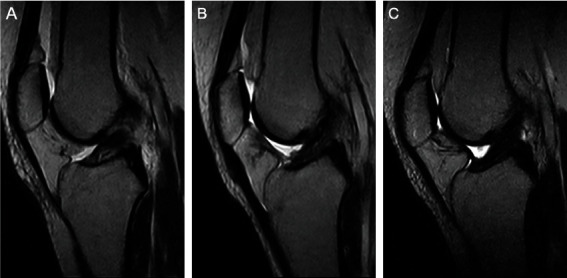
Pre- and postoperative MRI images of a patient treated with primary ACL repair using transosseous tunnel technique. (a) A sagittal T1-weighted MRI image of a proximal type I tear with excellent tissue quality is seen. (b) A sagittal T1-weighted MRI image at six months after surgery. (c) A sagittal T1-weighted MRI image at one year after surgery.
